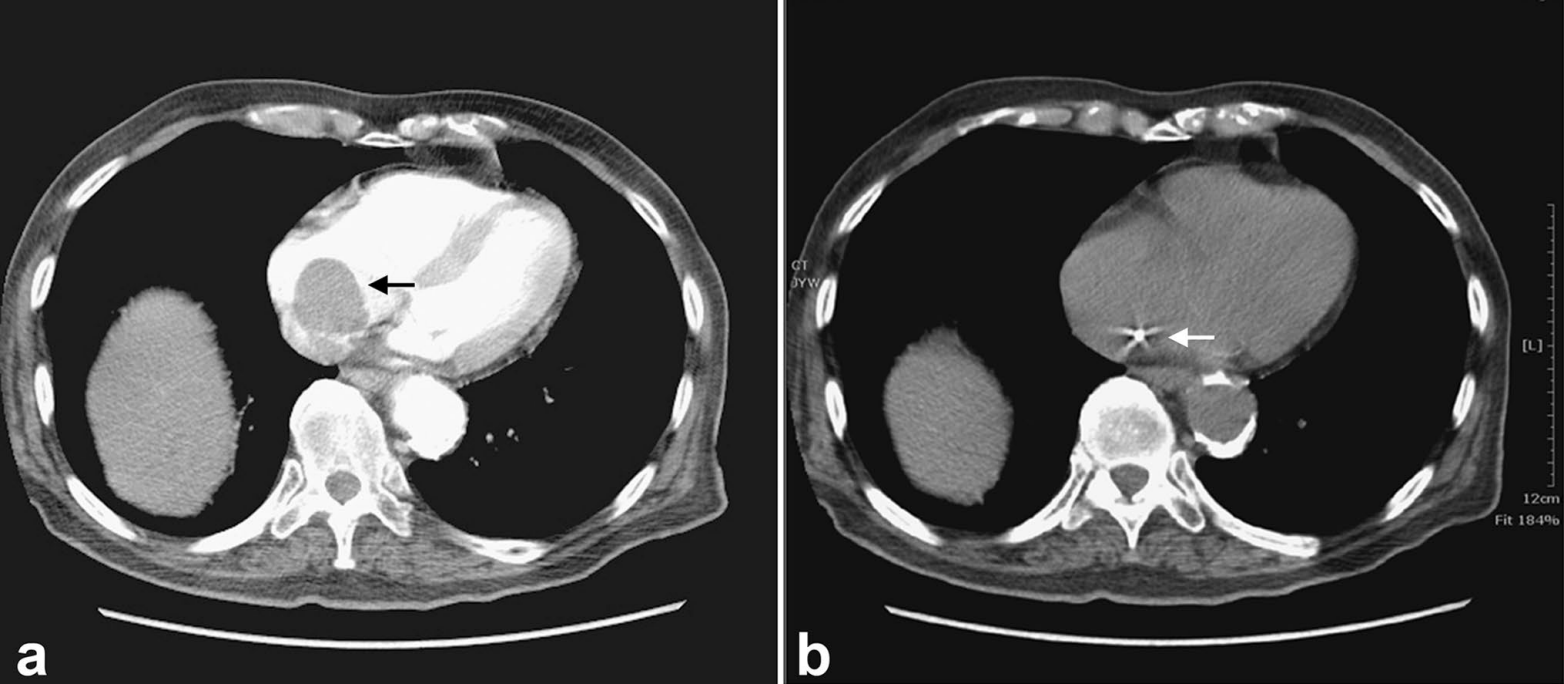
Computed tomographic views showing a space-occupying lesion popping up from the septum (black arrow) in the phase with contrast (a) and a pearl-like calcification (white arrow) in the phase without contrast (b)
